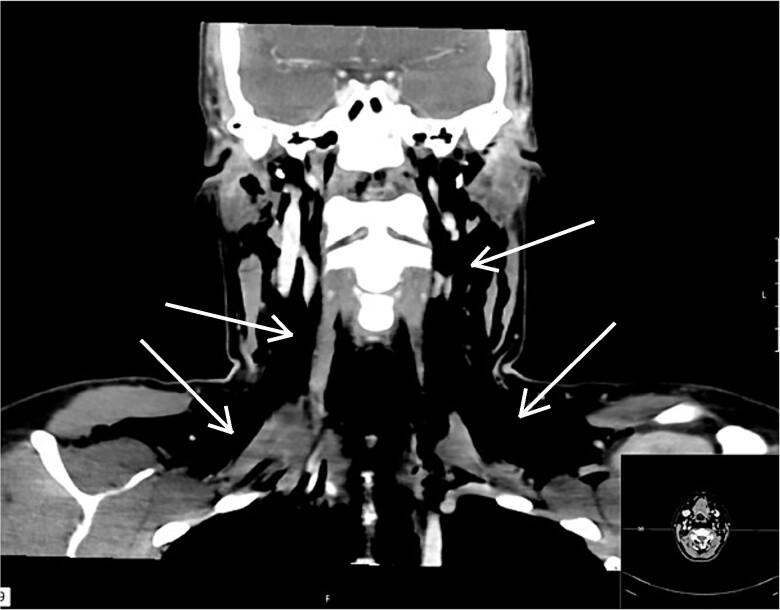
Frontal CT images of the collum. Arrows showing air pockets.
